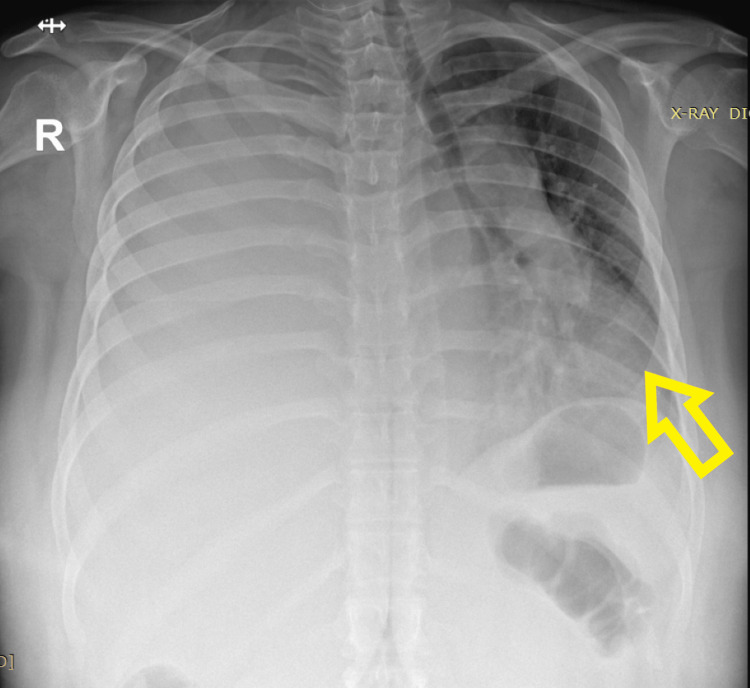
Chest X-ray at presentation Gross right-sided pleural effusion displacing mediastinal contents to the left side. The arrow shows the displaced mediastinal contents and tracheal deviation toward the left side.
